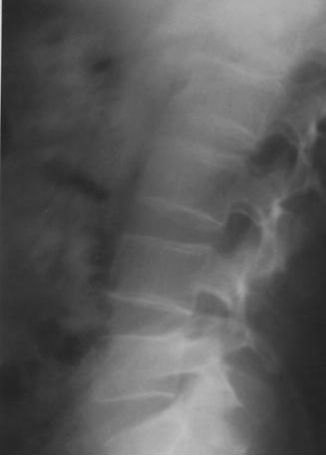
Radiography of lumbar vertebras before seizures showing bone demineralization (patient n 2).
